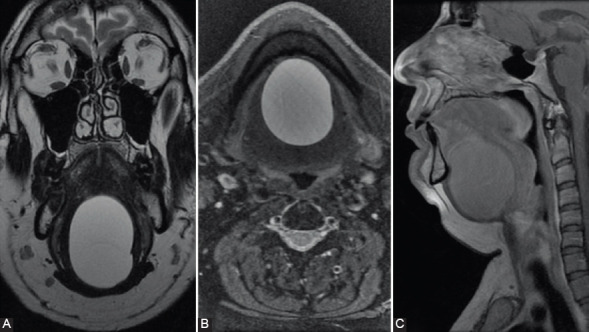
Magnetic resonance imaging of a patient with a lingual cyst with respiratory epithelium (LCRE) that demonstrates an approximately 6 cm cystic mass beneath the tongue in the coronal (A), axial (B), and sagittal planes (C). The lesion shows high signal on both basic (A) and fat-saturated T2-weighted (B) images, no contrast enhancement on T1 sequences (C). These aspects are in keeping with simple fluid collection. (A) COR T2 FSE; (B) AX FRFSE T2 Fat Sat; (C) SAG T1 FSE + contrast.
